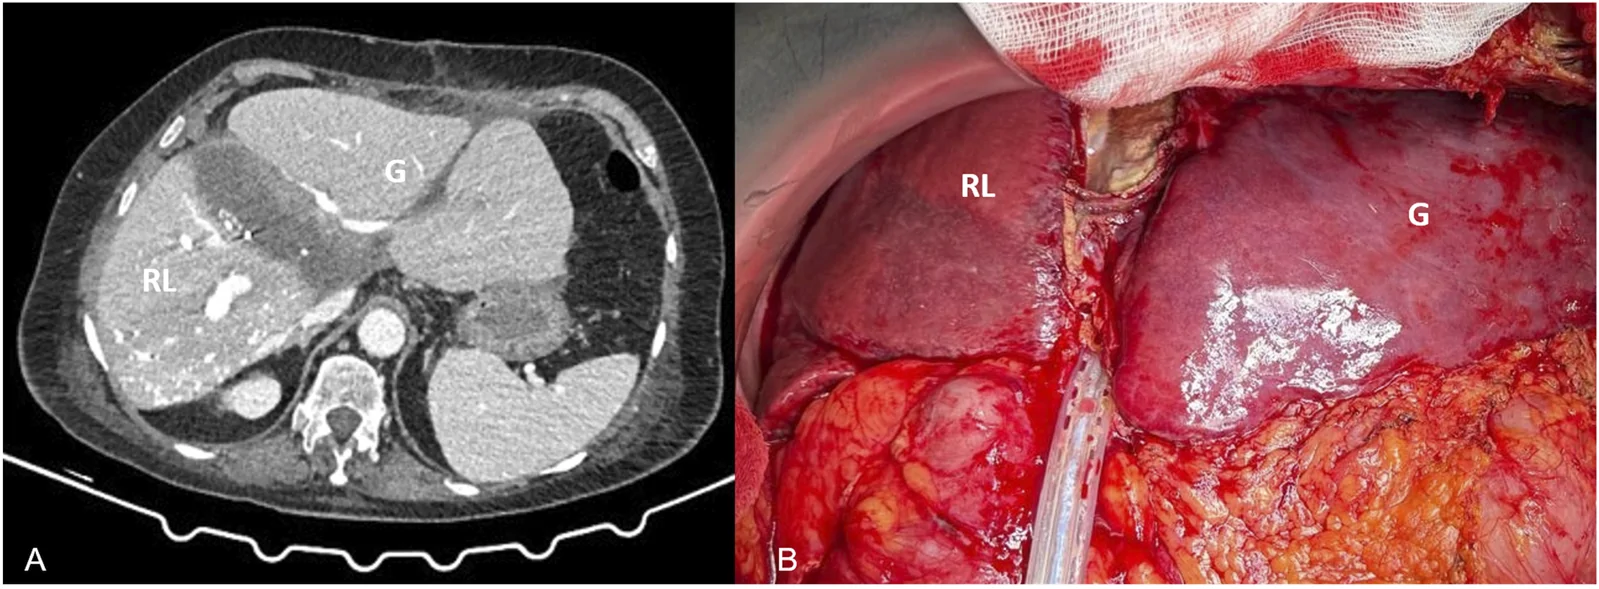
Perioperative imaging and intraoperative findings. (A) Postoperative contrast-enhanced CT scan after right portal vein embolization showing marked hypertrophy of the pediatric graft following stage-1 modified RAPID transplantation. (B) Intraoperative view of the second stage before completion right hepatectomy. RL, right native liver; G, pediatric graft.
